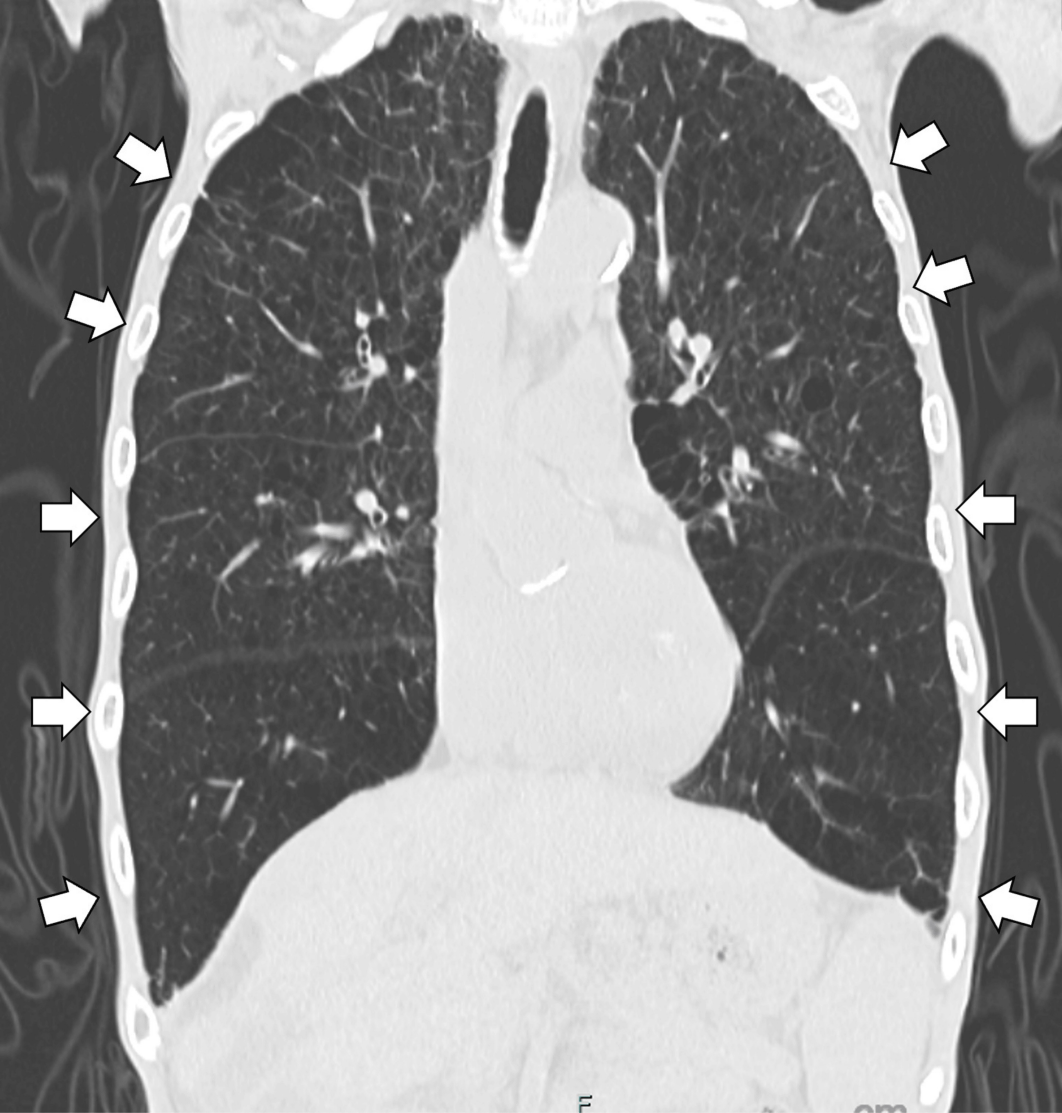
Chest computed tomography revealing emphysematous changes in both lungs, consistent with chronic obstructive pulmonary disease (white arrows).
